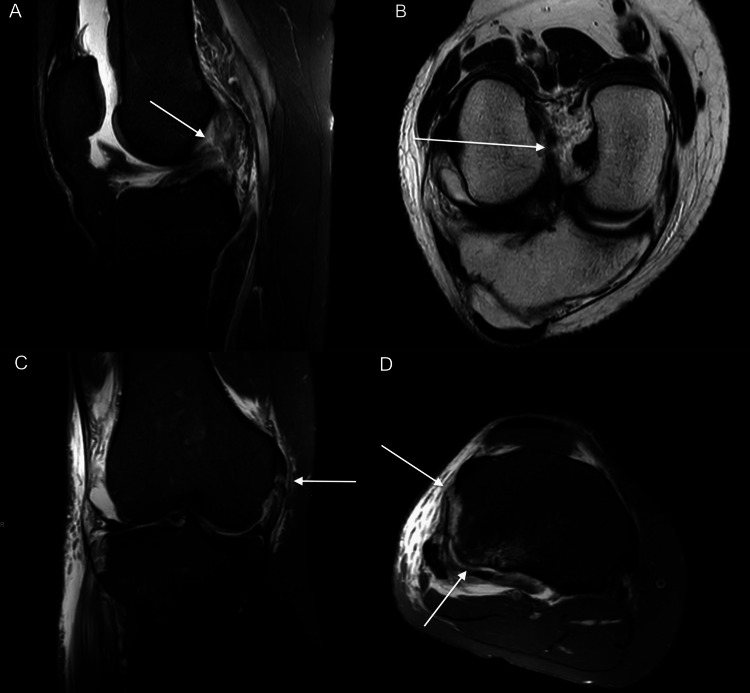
(A) Sagittal fat-suppressed proton density-weighted image and (B) paracoronal T2-weighted image show a total rupture of the ACL (arrows). (C) Coronal fat-suppressed proton density-weighted image demonstrates a partial rupture of the MCL (arrow). (D) Axial fat-suppressed proton density-weighted image shows avulsion of the anterolateral capsular structures involving the anterolateral ligament as well as a posterolateral impression fracture of the tibial plateau (arrows). No injuries to the meniscus were observed. ACL: anterior cruciate ligament, MCL: medial collateral ligament
